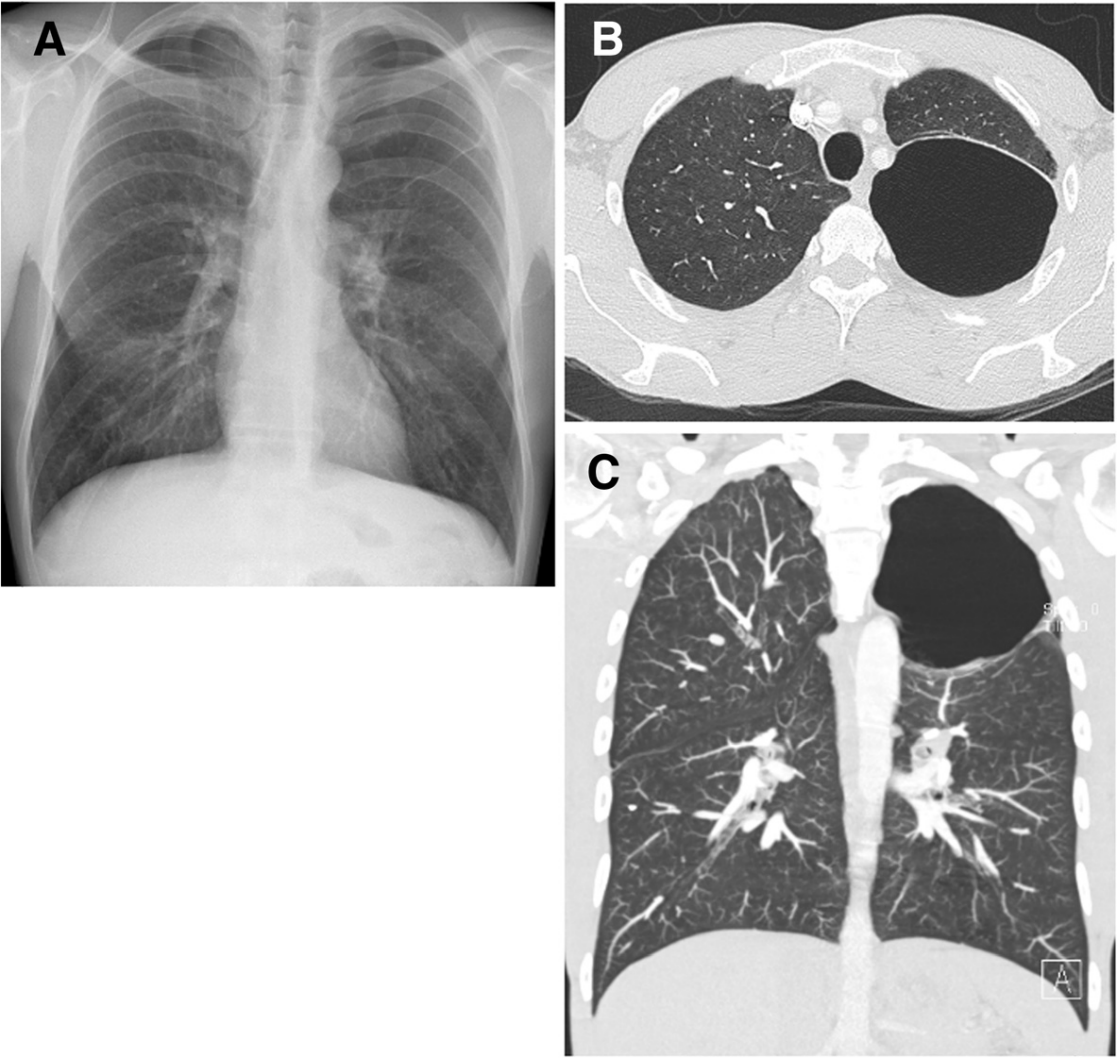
Initial chest radiograph of the patient. (PA view) showing a giant bulla (increased hyperlucent zone) of the left apex (A). HRCT showing axial (B) and sagittal (C) image (lung window) with a big lucent area without real definable walls.
